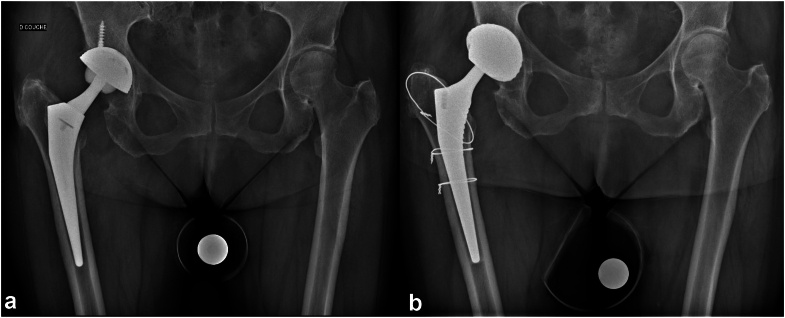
Extended limited femorotomy. (a) Preoperative radiograph of a patient with right prosthetic hip infection. (b) Postoperative radiograph of a stem extraction with limited extended femorotomy and reimplantation of a standard stem with 3 wires.
